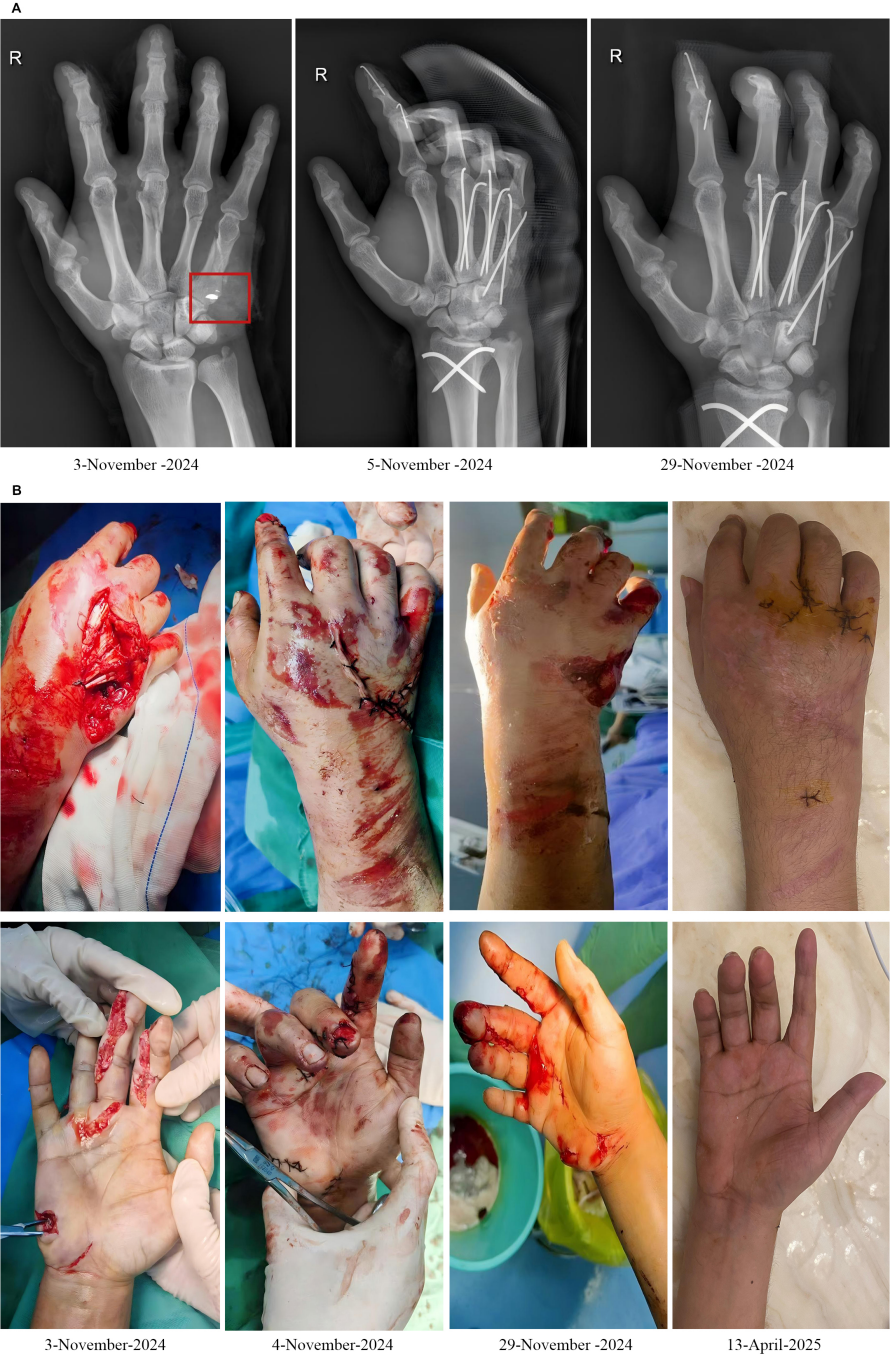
Radiographic images of the patient’s hand (A). Photographs of the patient’s wound debridement, suturing, suture removal, and wound healing progress (B).
